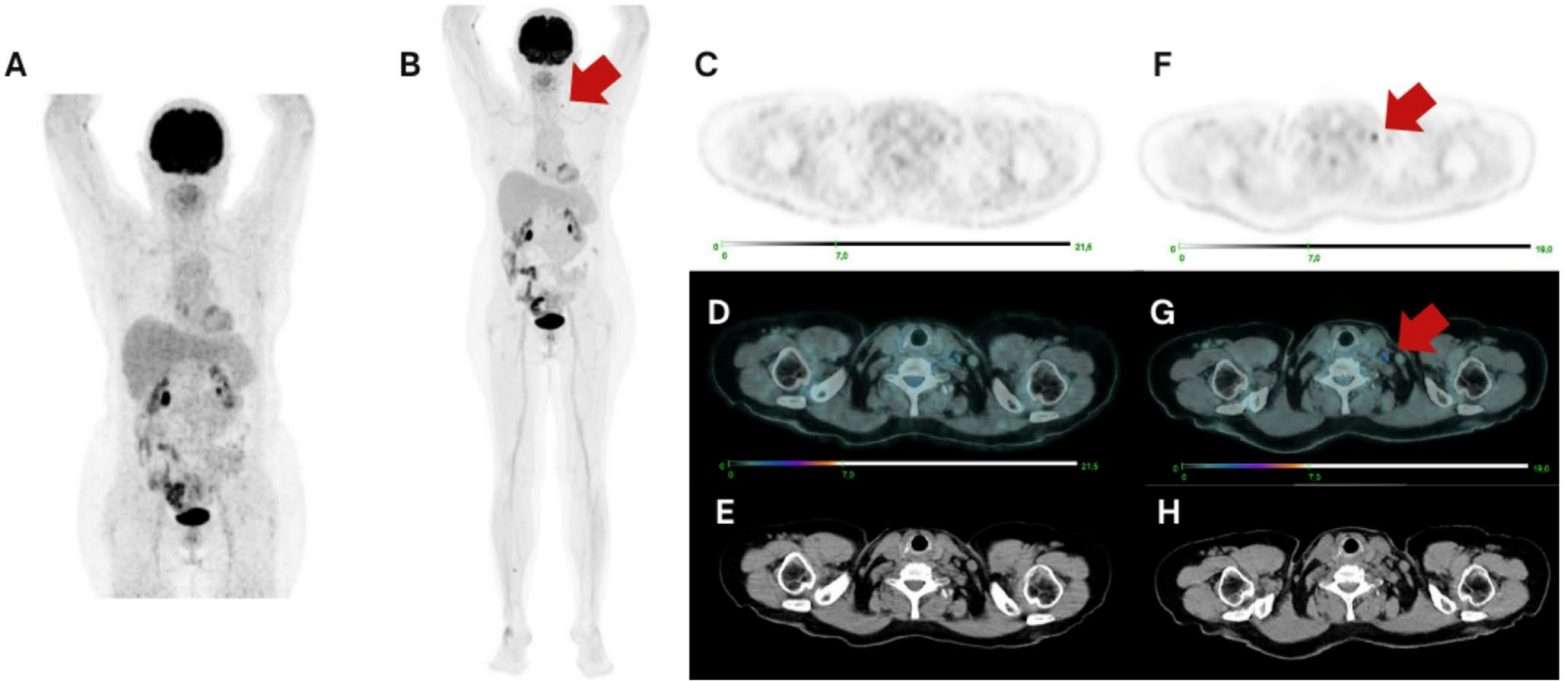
[18 F]FDG PET/CT performed for restaging in a patient with advanced high-grade ovarian cancer, with previous peritoneal localizations, currently undergoing treatment with niraparib. The SAFOV examination is negative (A, C-E), while the LAFOV PET/CT reveals low level uptake in a small left supraclavicular lymph node (B, F-H). Biopsy of this lymph node confirmed metastatic ovarian carcinoma
